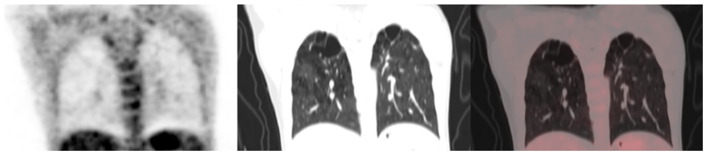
A 40-year-old female status post-treatment for drug-sensitive pulmonary tuberculosis. She is HIV negative. From left to right, coronal section of the PET, CT, and fused PET/CT images are shown. Bilateral fibrocavitory changes seen in the upper lobes show no significant residual metabolic activity.
